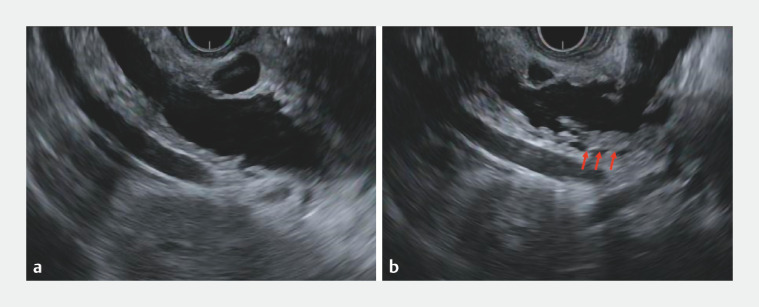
Endoscopic ultrasonography revealing pancreatic duct dilation and pancreatic cyst with a suspected mural nodule connected with the main pancreatic duct (red arrow).
